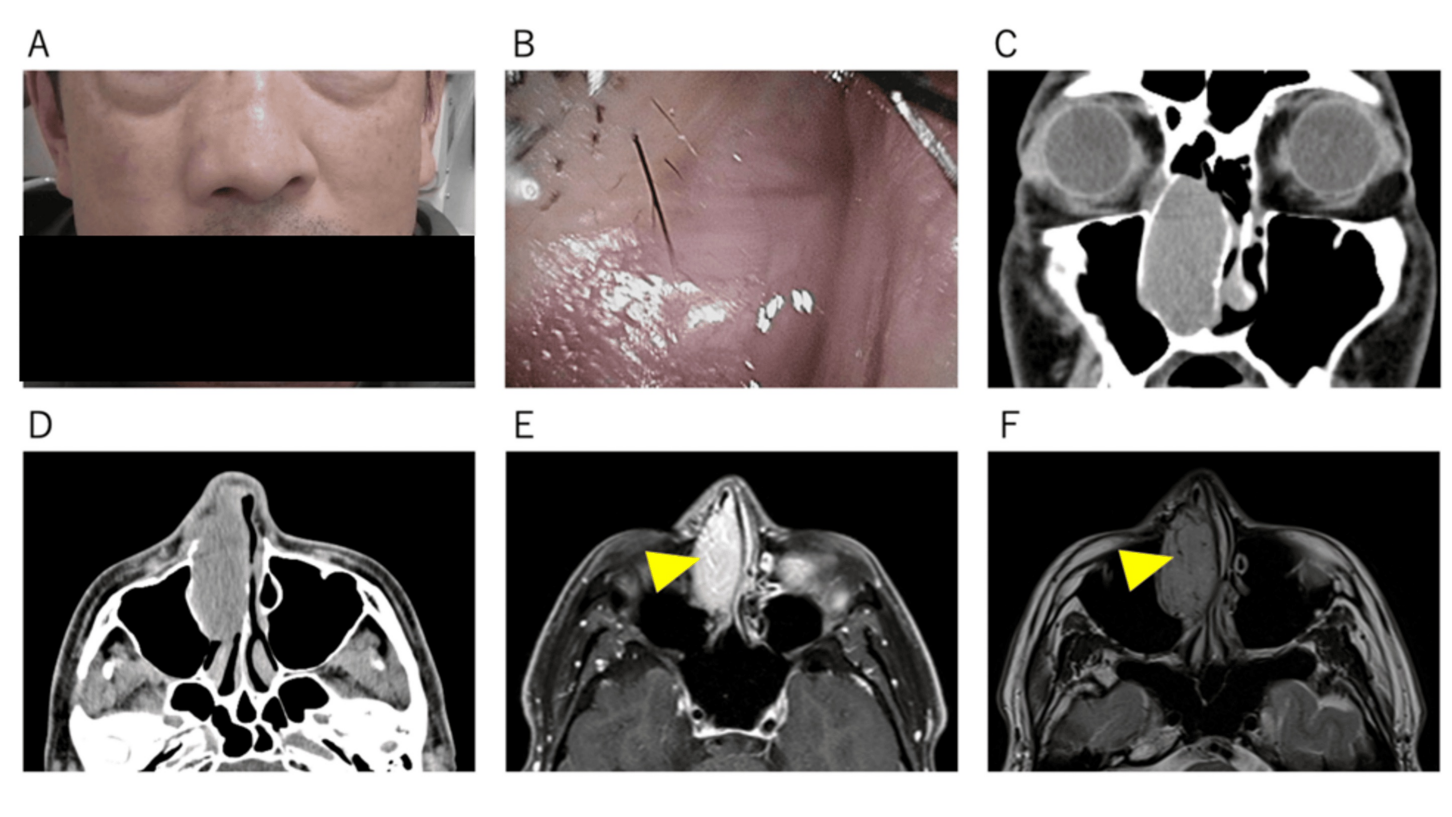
Initial Clinical Findings at Presentation (A) Slight swelling of the right nasal ala and the right side of the nasal root is observed. (B) The right anterior nasal cavity is completely filled by a smooth-surfaced mass. (C-D) CT images show that the tumor occupies the nasal cavity posteriorly without evidence of bony destruction. (E) Gadolinium-enhanced T1-weighted MRI demonstrates marked enhancement with internal tubular structures (yellow arrowhead). (F) On T2-weighted imaging, the tumor appears isointense with internal flow voids (yellow arrowhead). CT, computed tomography; MRI, magnetic resonance imaging
